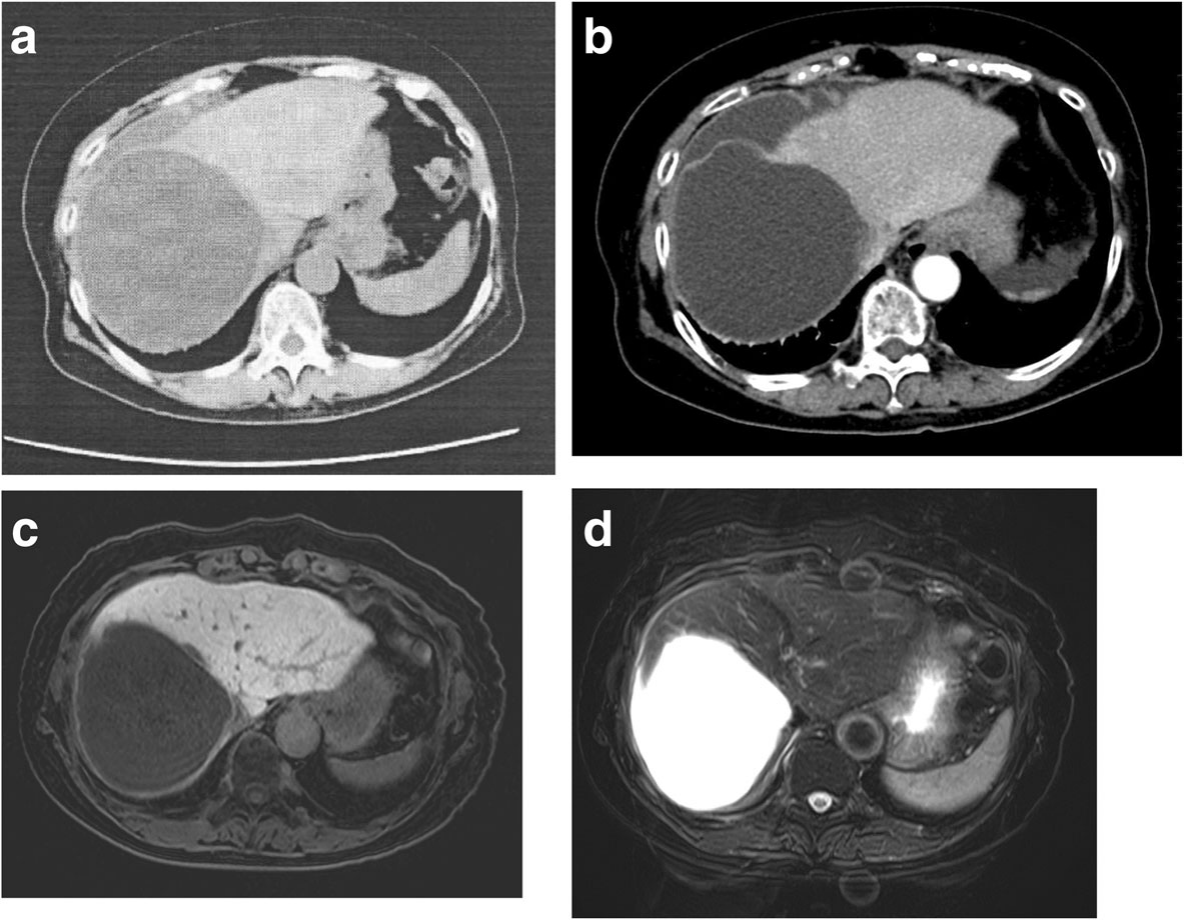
a Computed tomography (CT) scan before admission to hospital. b CT scan during the examination. c Magnetic resonance imaging (MRI) T1-weighted image. d MRI T2-weighted image
